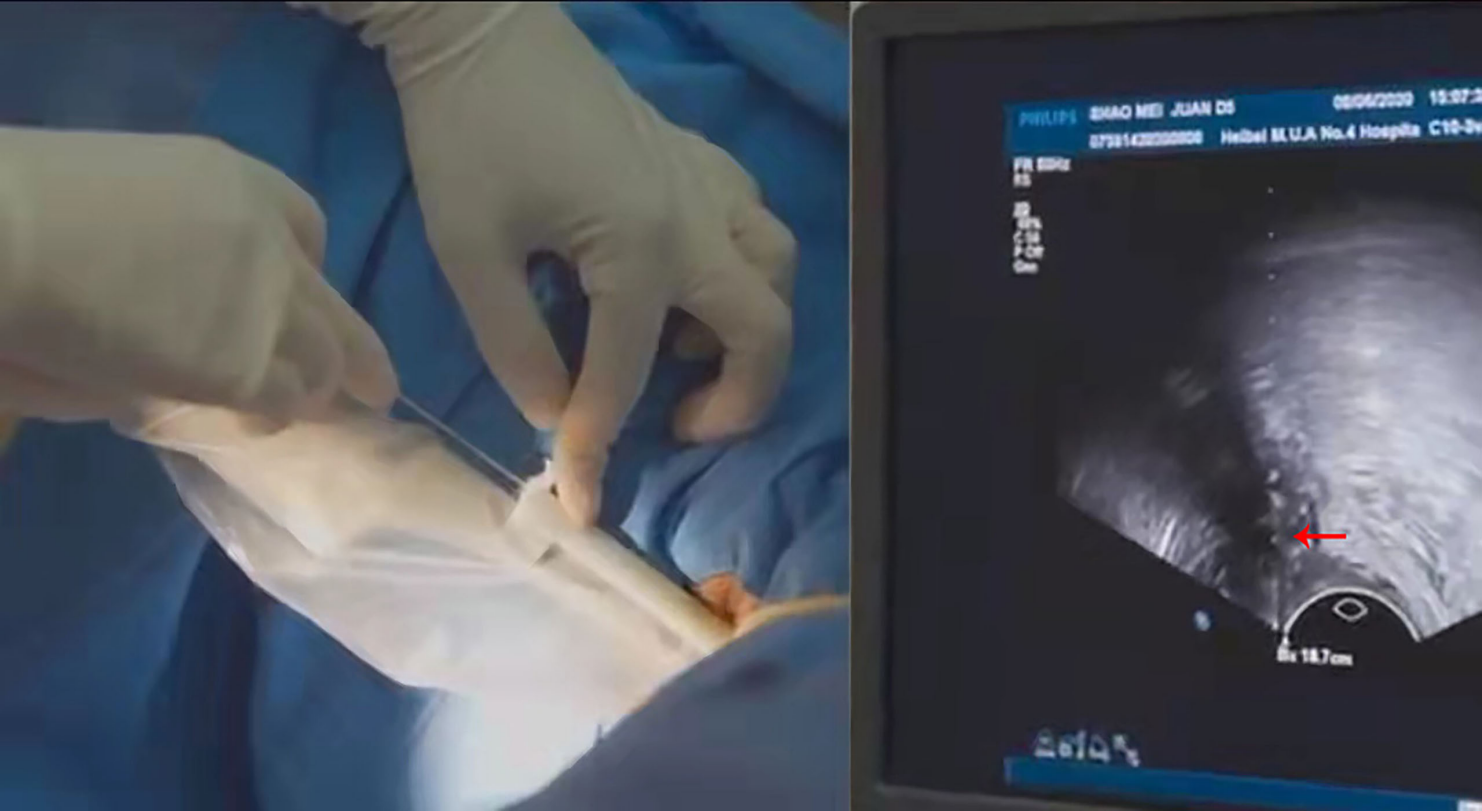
Implant therapy with the puncture bracket under transvaginal ultrasound guidance.
